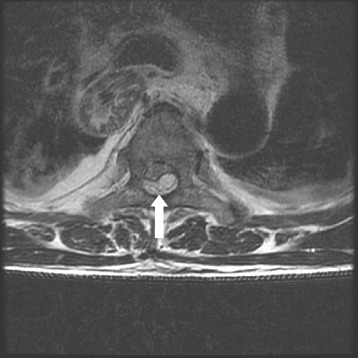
T2-weighted axial magnetic resonance image at the Th5 level. The spinal epidural hematoma (*arrow*) is observed to be pressing the spinal cord toward the ventral side
